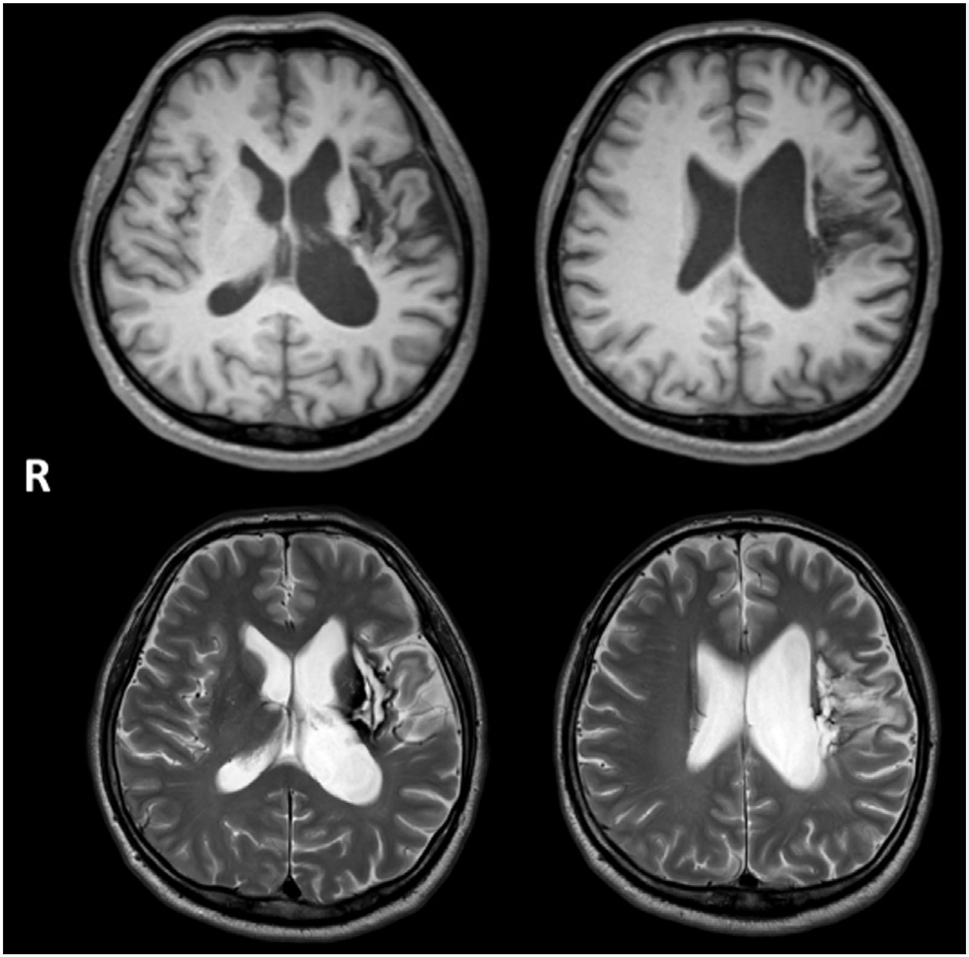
Axial MRI images showing the lesion resulting from a left putaminal hemorrhage, which had spread to thalamus and subcortical regions of the frontotemporal lobe. Upper and lower images show T1- and T2-weighted images, respectively.
